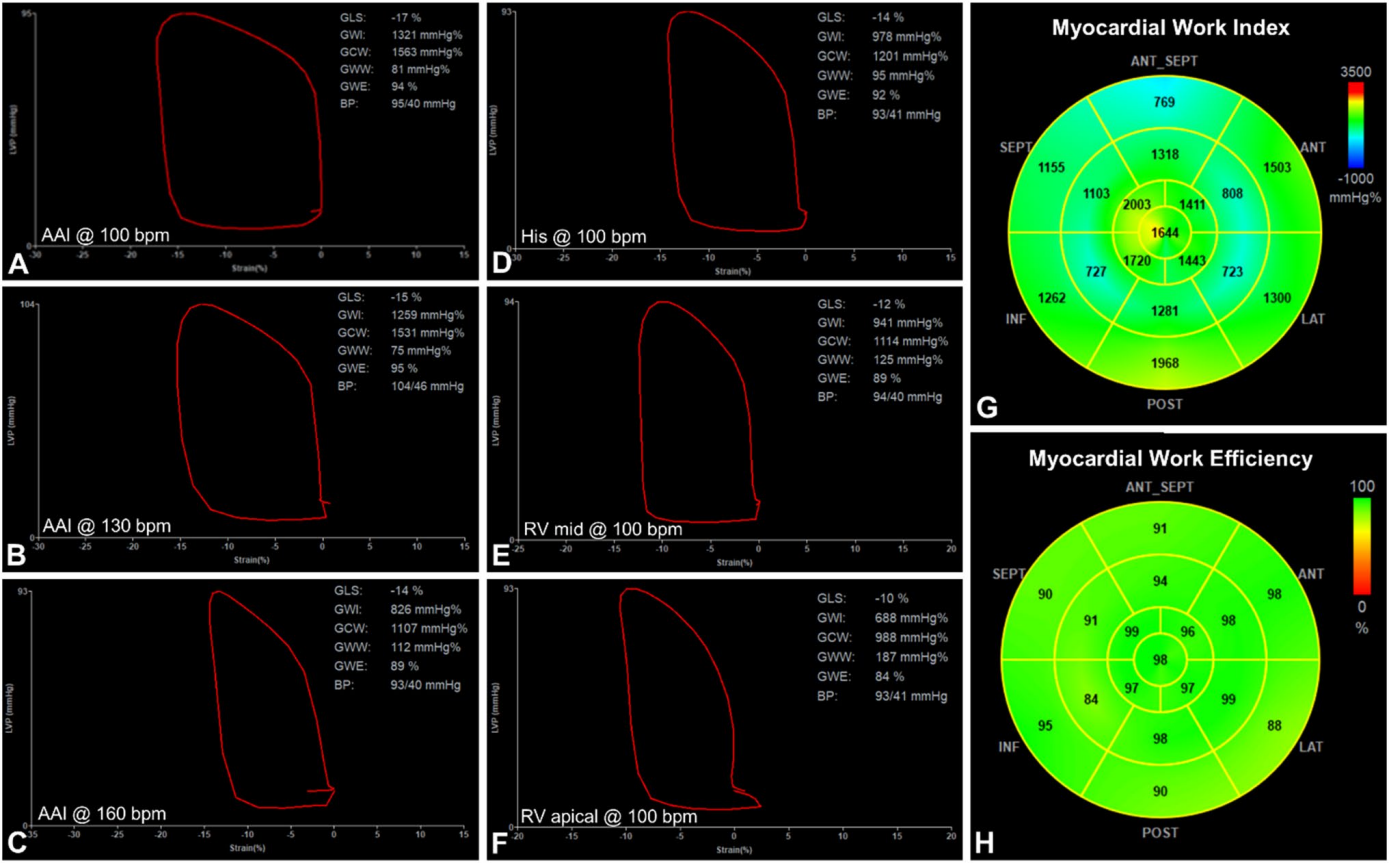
Example case of non-invasively derived pressure-strain loop and derived myocardial work indices from echocardiography. A-C AAI pacing was performed at increasing heart rates, demonstrating decreased global work index (GWI), global constructive work (GCW), and global work efficiency (GWE) with increasing global wasted work (GWW) at increased rates. D-F Similar trends where seen with differences in pacing site, from His bundle, to right ventricular (RV) mid-ventricle to RV apical pacing. Examples of regional derived myocardial work index (G) and myocardial work efficiency (H) are displayed from the same patient during AAI pacing at 100 bpm. BP blood pressure, GLS global longitudinal strain
